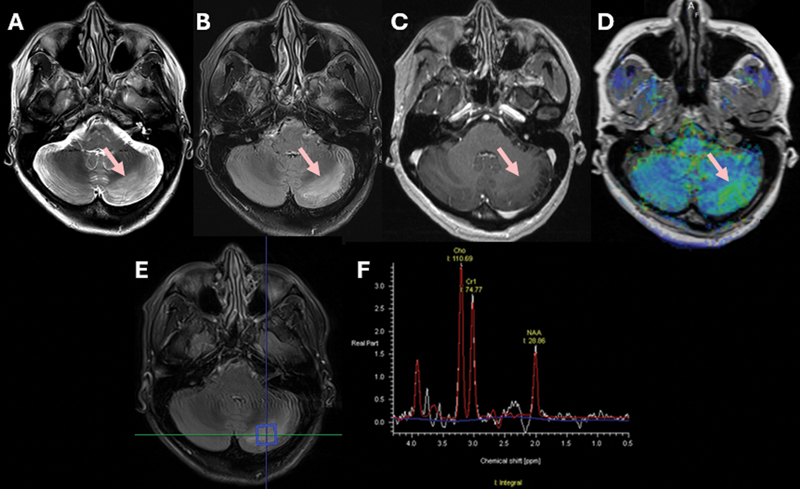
One-week follow-up brain MRI scan. (
